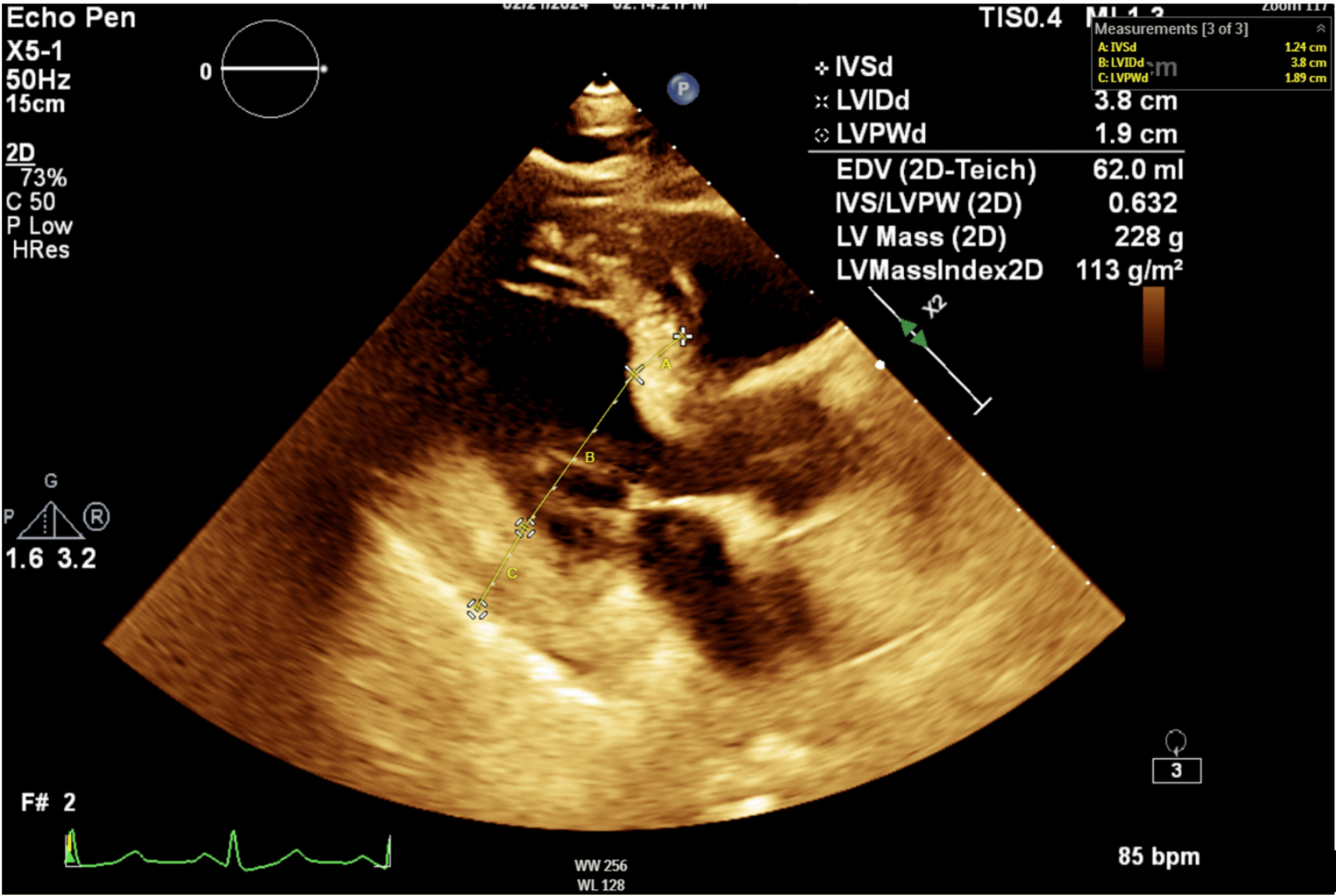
Transthoracic echocardiography parasternal long axis view showing mild left ventricular hypertrophy.
